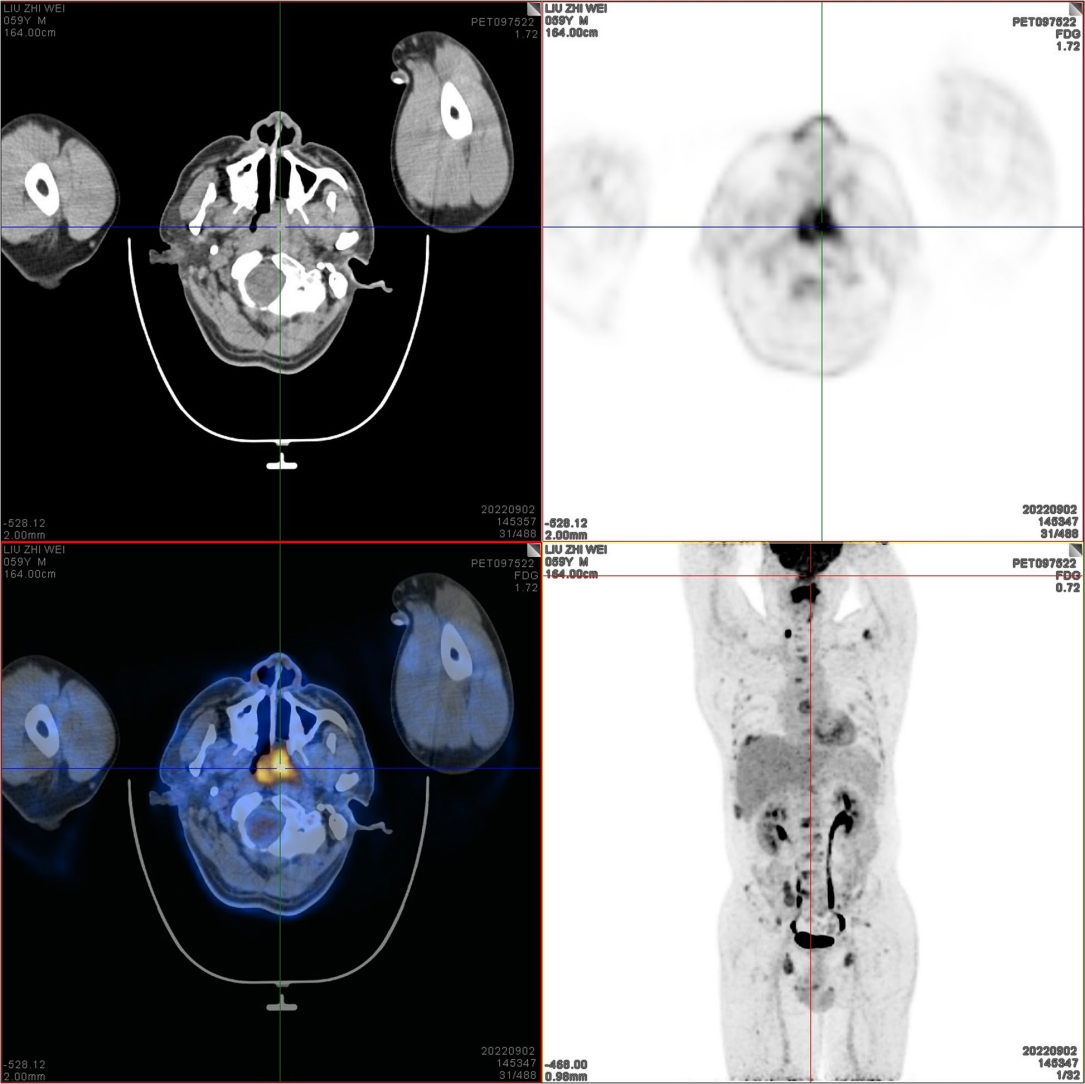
PET-CT scan findings of the loss of left pharyngeal recess and a palpable mass formed by incrassated soft tissue on posterior and left walls of the nasopharynx, of which the FDG metabolism increased
